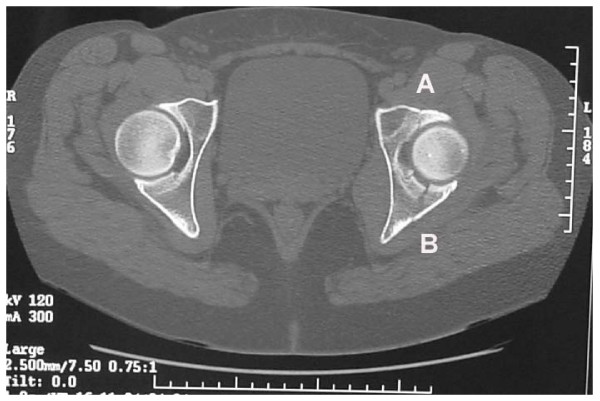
CT scan of the hip. Note the fracture line involving the anterior column (A) and the posterior column (B).
